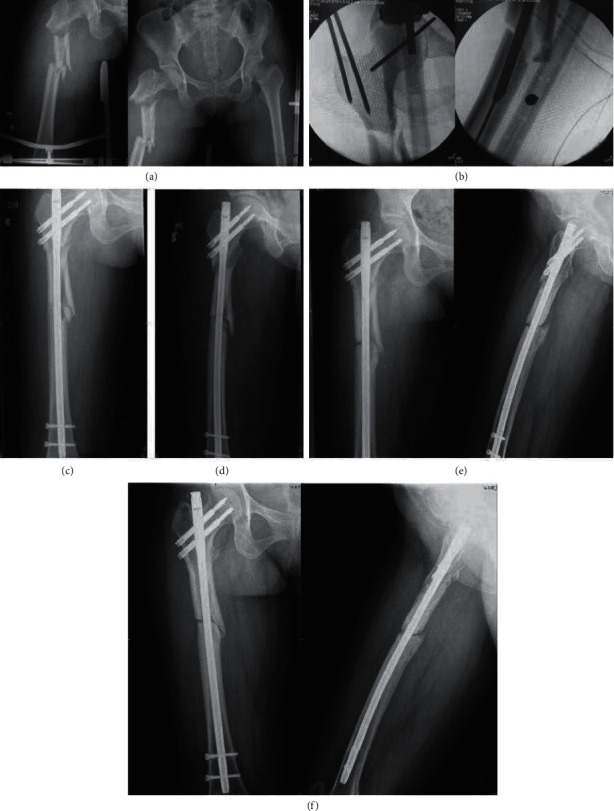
Preoperative X-ray of the patient, Seinsheime classification IV (a). During the operation, the length of the fracture was restored by traction with the retractor. Then, closed reduction was used during the operation. The gold finger was used to pass through the broken end of the fracture, and the full length intramedullary nail was fixed (b, c). Two months after the operation, the position of internal fixation was good, and there was no sign of fracture healing (d). Six months after the operation, X-ray examination showed that the proximal fracture line was blurred, and the distal fracture line was still clearly visible (e). Twelve months after the operation, X-ray examination showed that the proximal fracture line disappeared, the distal fracture line was clear, and the broken end of the fracture hardened and was diagnosed as atrophic nonunion (f).
